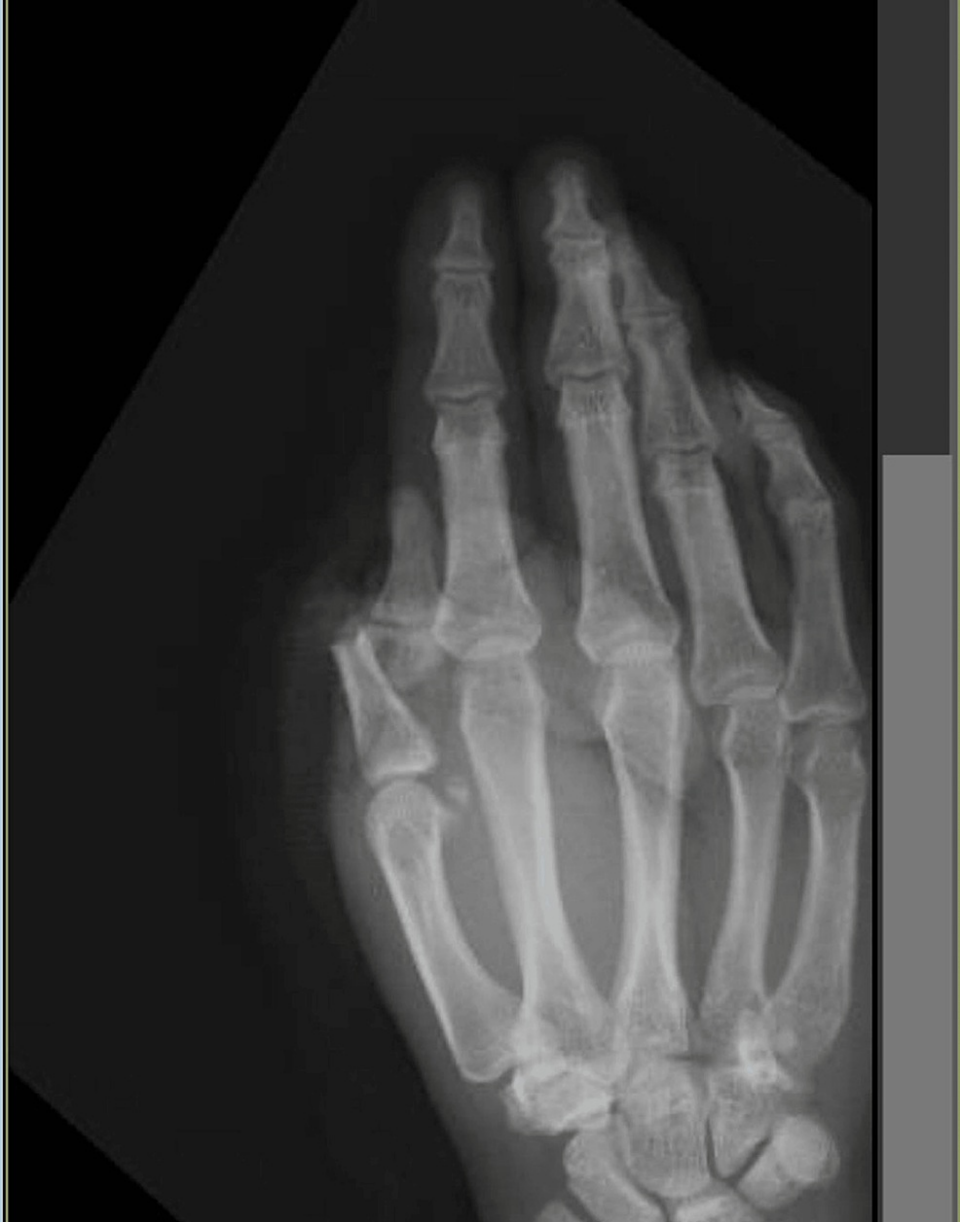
Posteroanterior view of the X-ray of the right hand showing displaced fracture through the neck of the proximal phalanx of the thumb.
